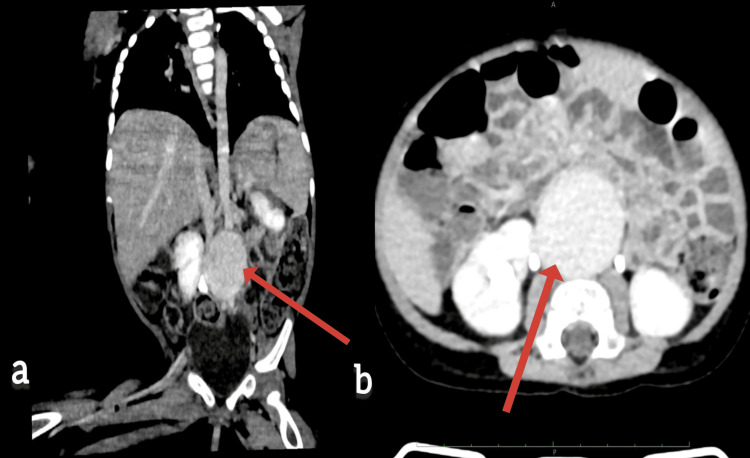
Contrast-enhanced computed tomography (CECT) showing an infrarenal aneurysm.
